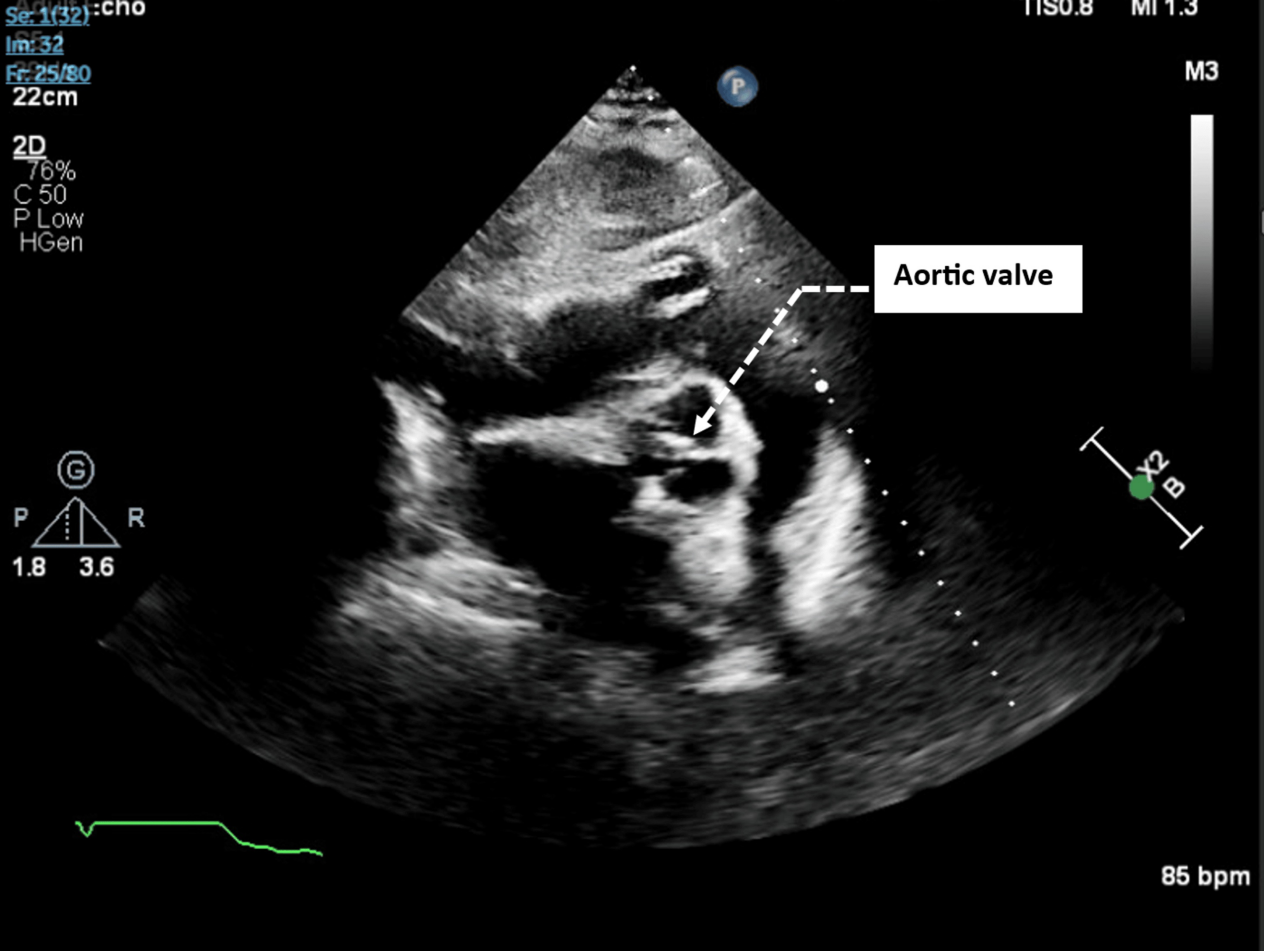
A transthoracic echocardiogram obtained at presentation to the hospital shows a seemingly normal aortic valve, with no visible vegetations.
